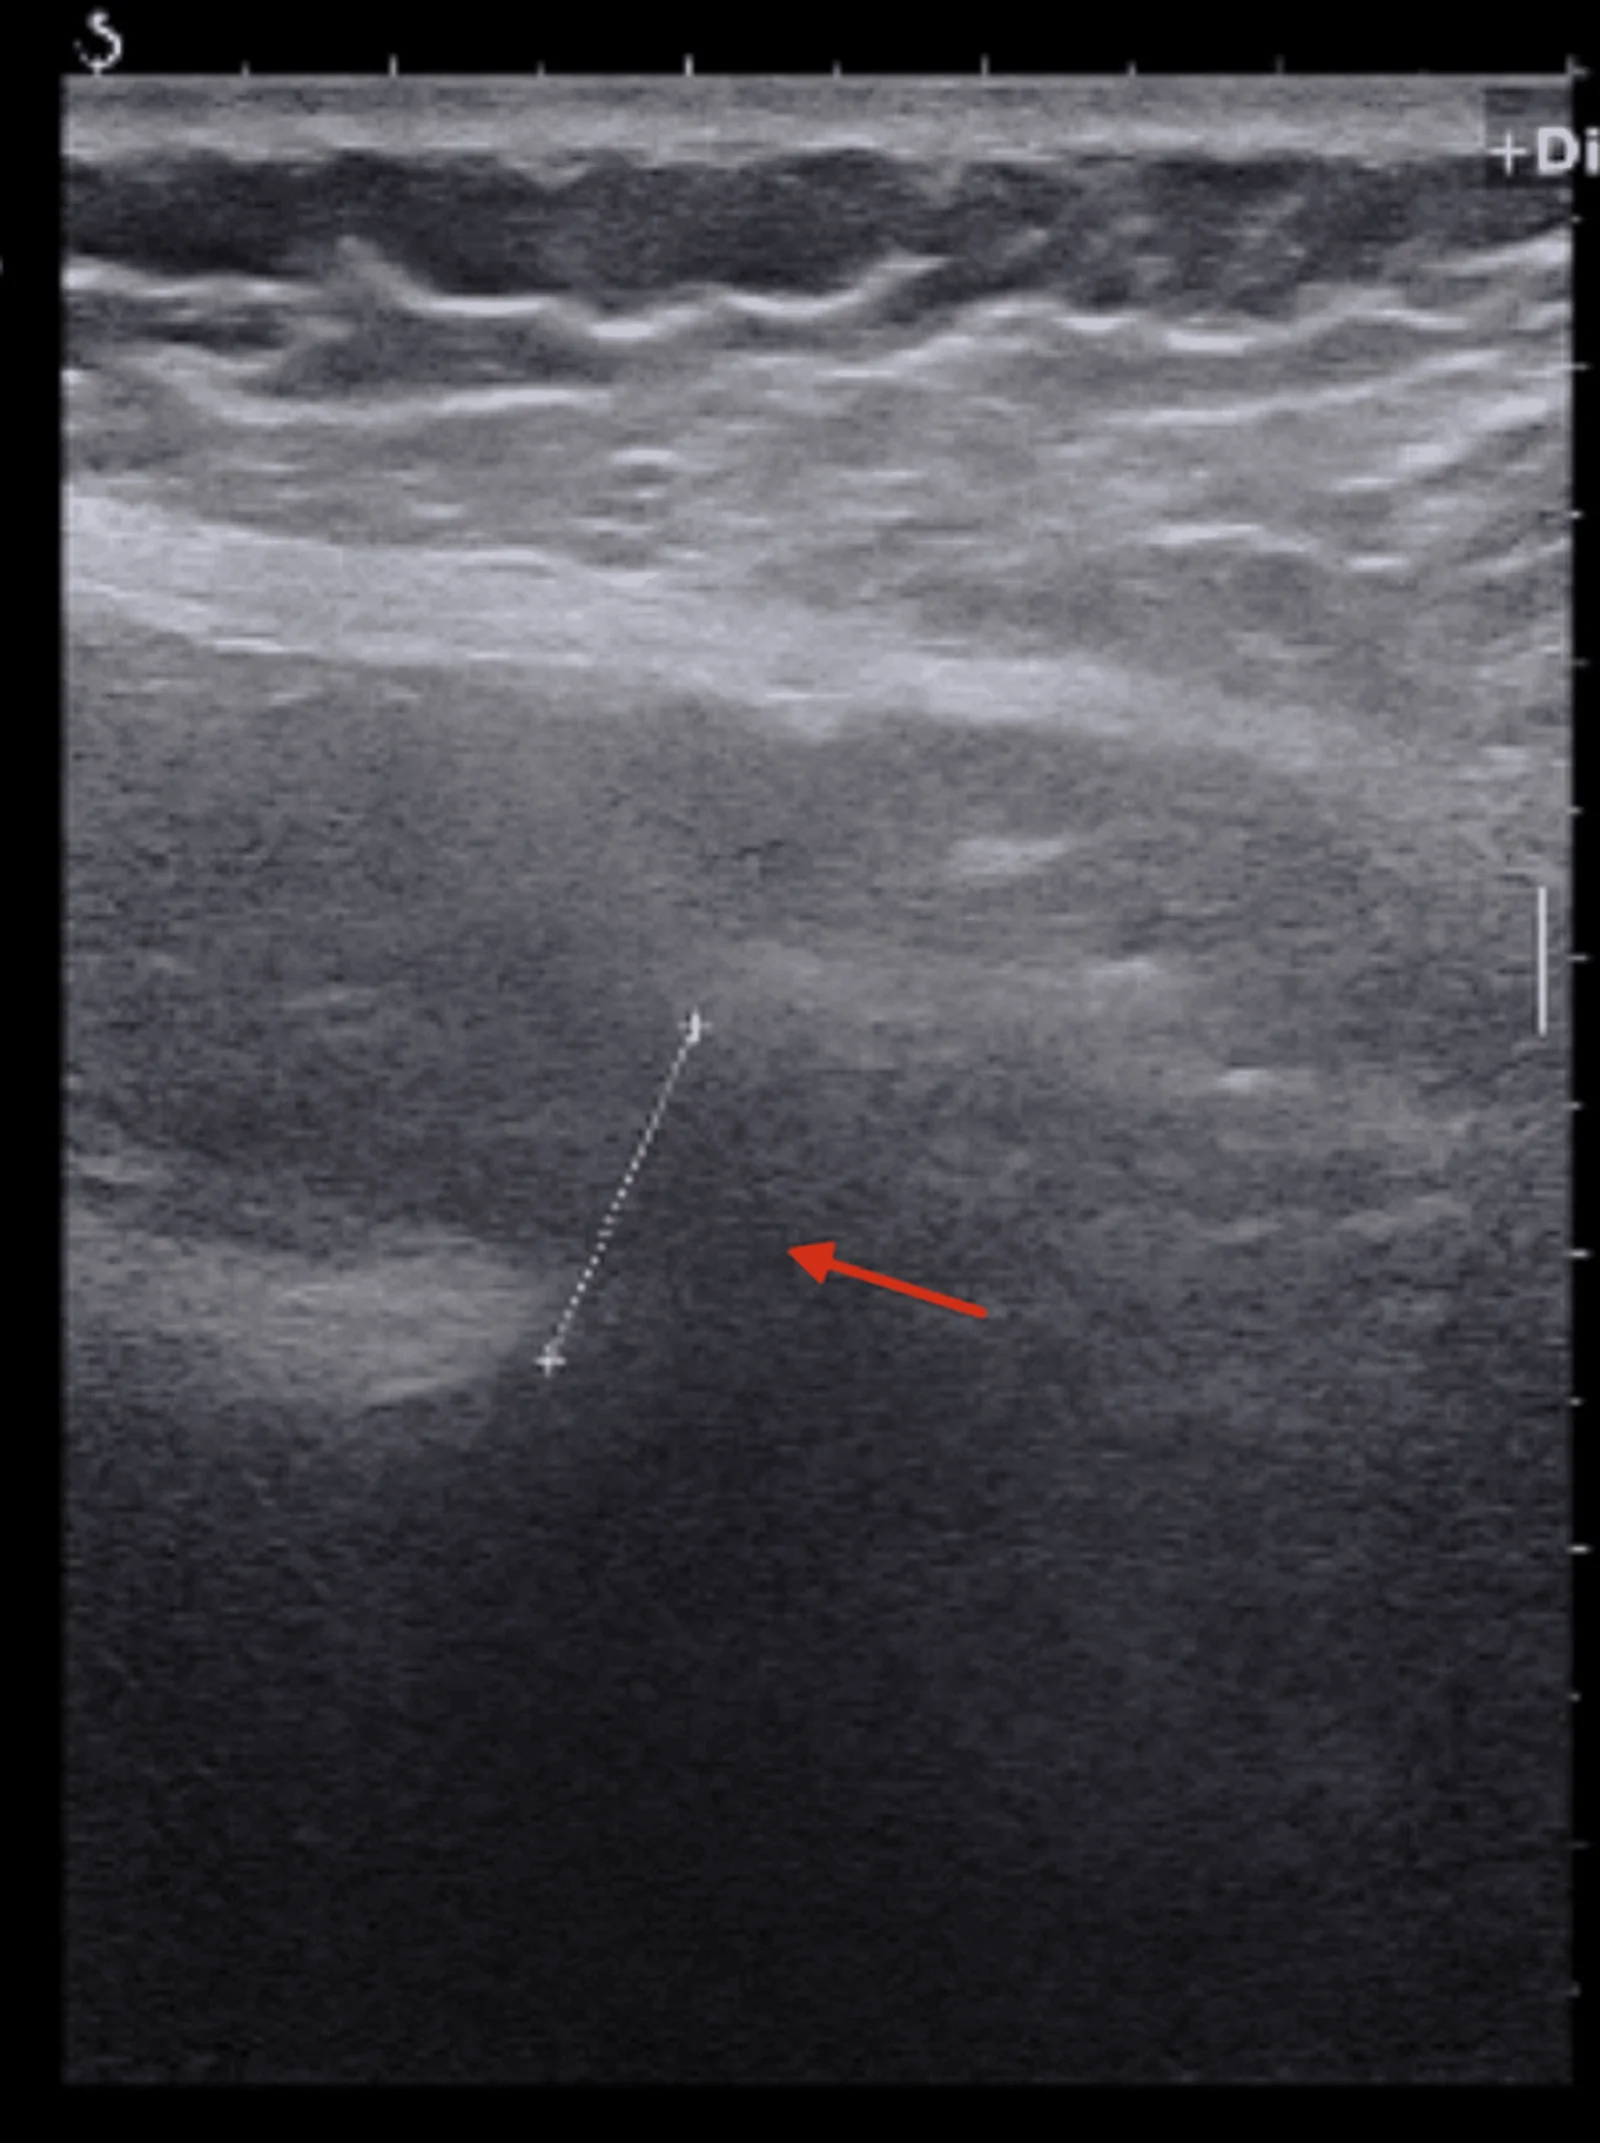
Abdominal ultrasound A 4-cm defect within the left superior lumbar triangle (arrow), associated with protrusion of fatty tissue through the abdominal wall, forming a hernia sac measuring approximately 7 × 2 cm.
